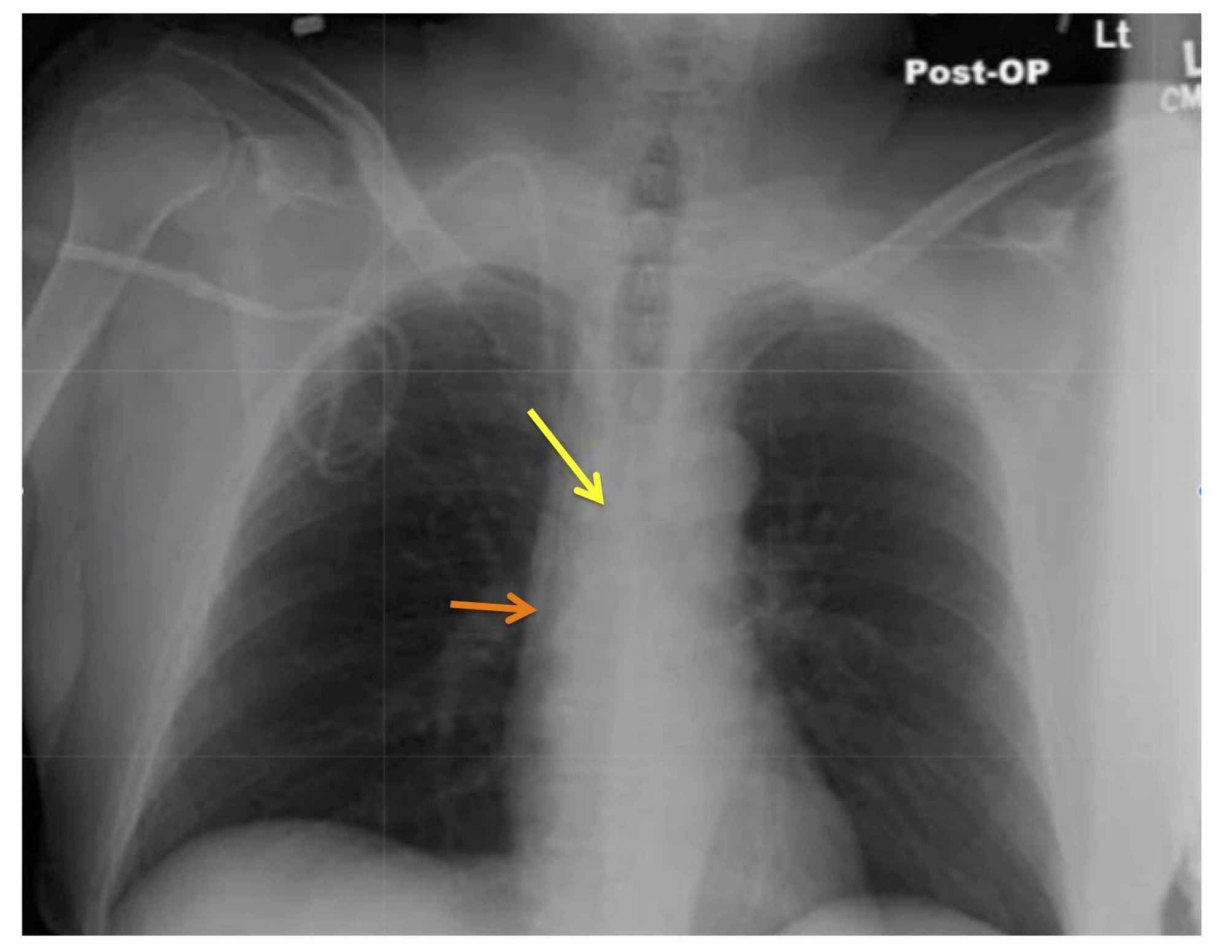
Chest radiograph showing a central line This is a right-sided central line in internal jugular vein (the tip is pointed out by the orange arrow). The central line is positioned near the right tracheobronchial angle (pointed out by the yellow arrow) [3].
